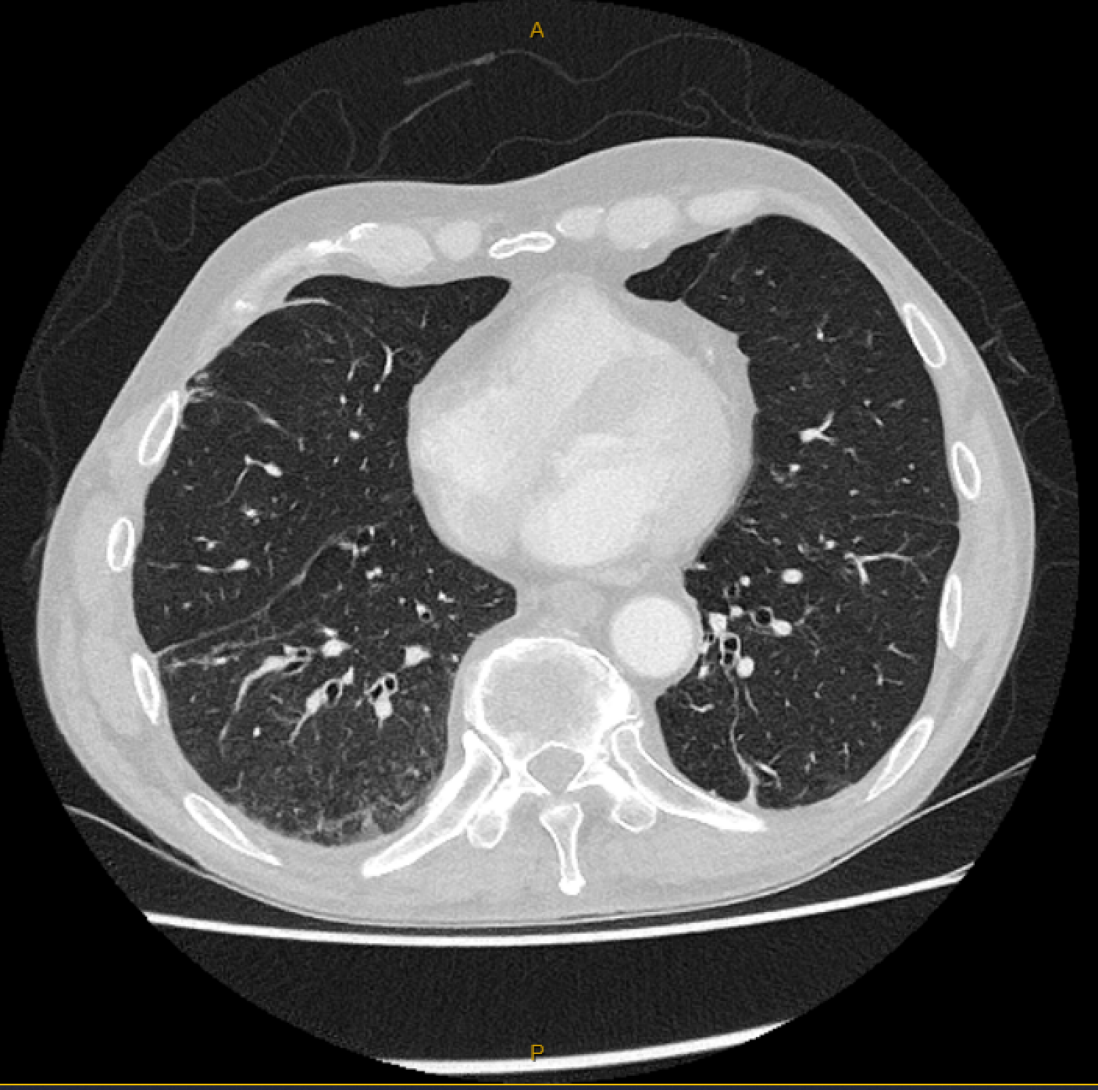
Axial computed tomography (CT) Chest after 6 months demonstrating resolution of majority of earlier imaging findings.
